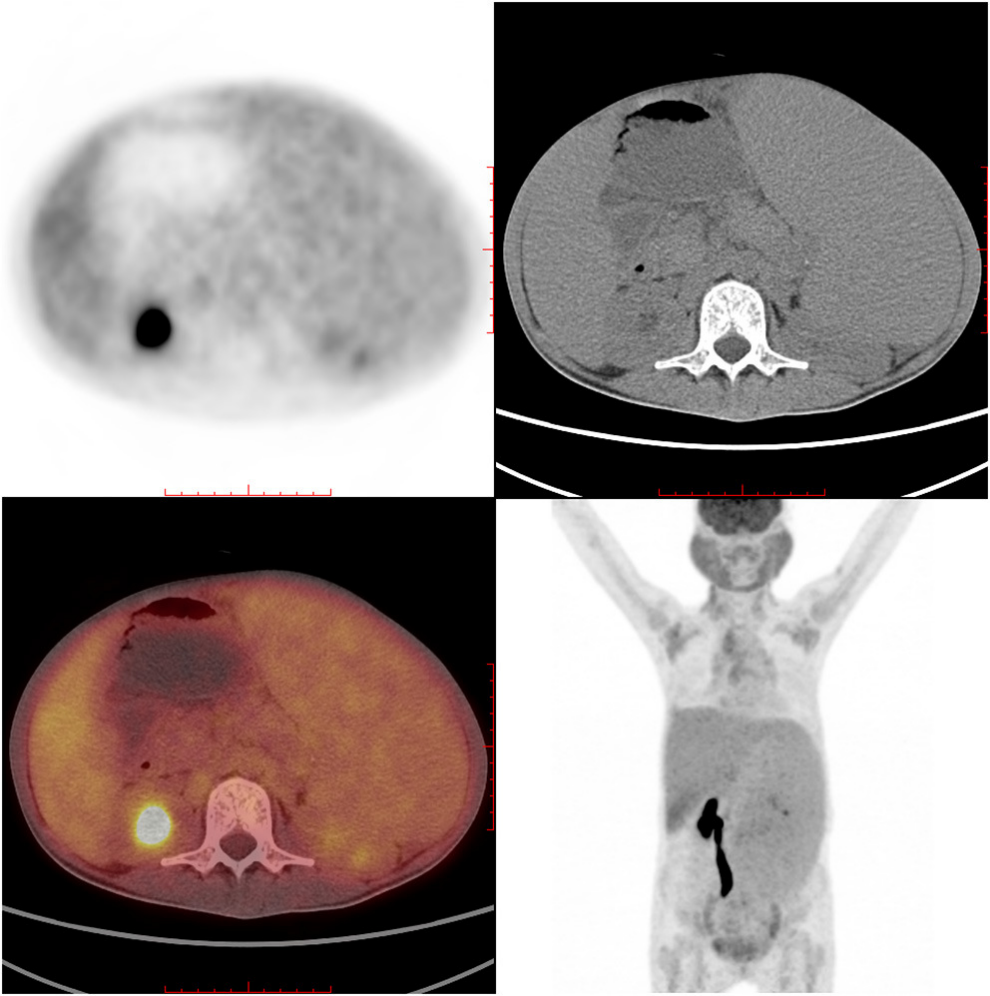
PET-CT showed high glucose intake in salivary glands, lymph nodes, liver, and spleen.
